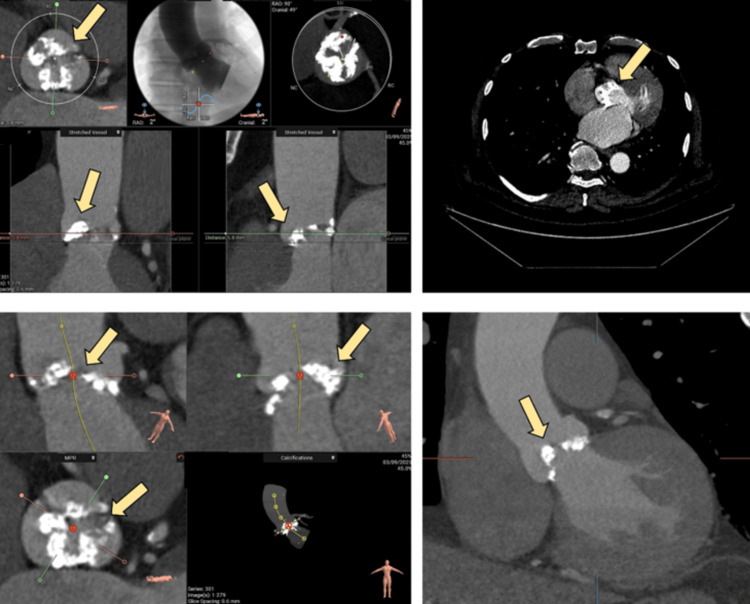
Preprocedural computed tomography demonstrating extensive aortic valve calcification. Multiplanar reconstruction images of the aortic root and aortic valve complex show massive leaflet and annular calcification (arrows). The extensive and asymmetric calcium burden resulted in a critically stenotic valve orifice and represented the principal anatomical challenge for transcatheter valve delivery.
